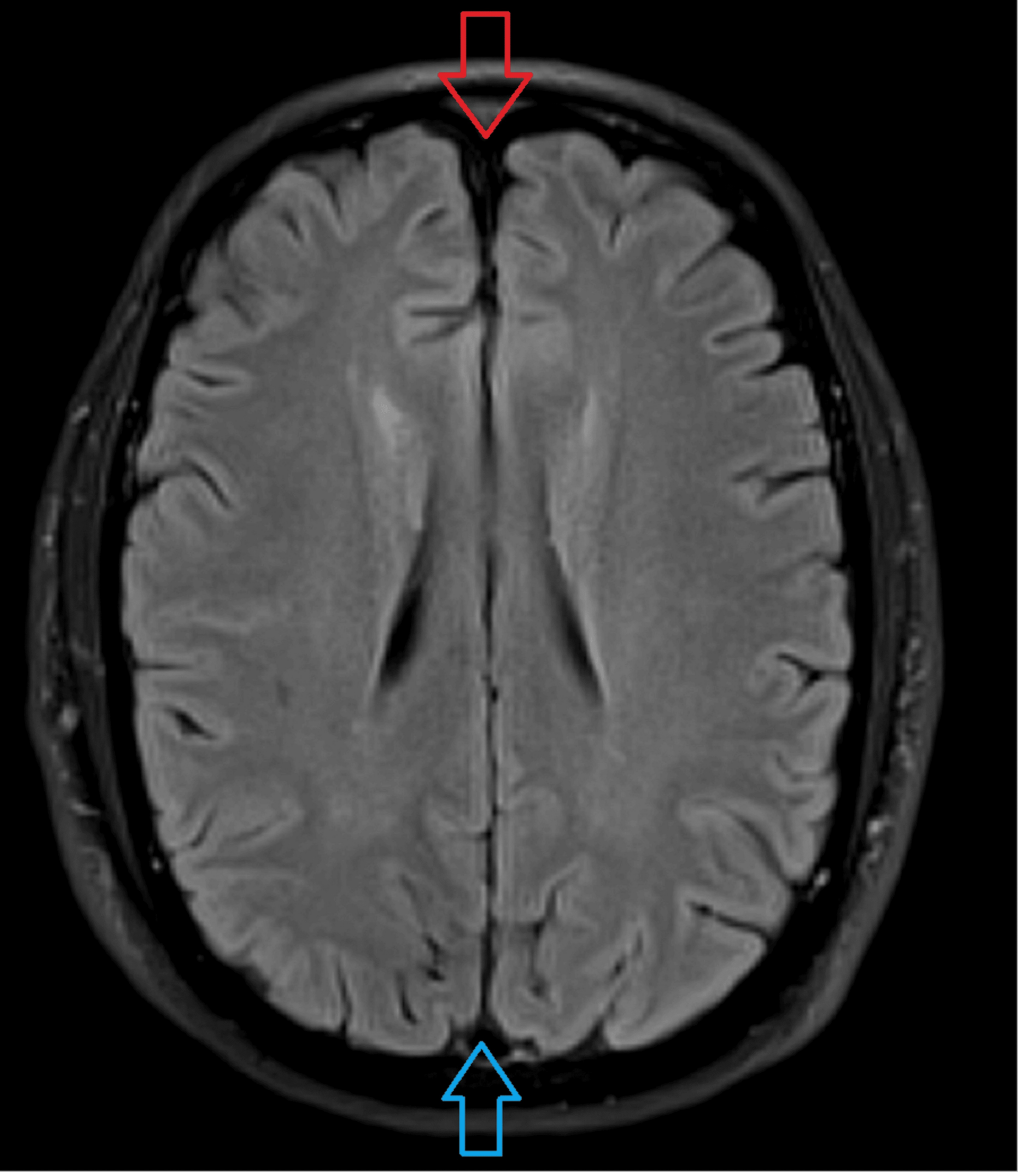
Axial MRI of the brain No significant neocortical atrophy seen in the frontal (red arrow) and parietal lobes (blue arrow).
